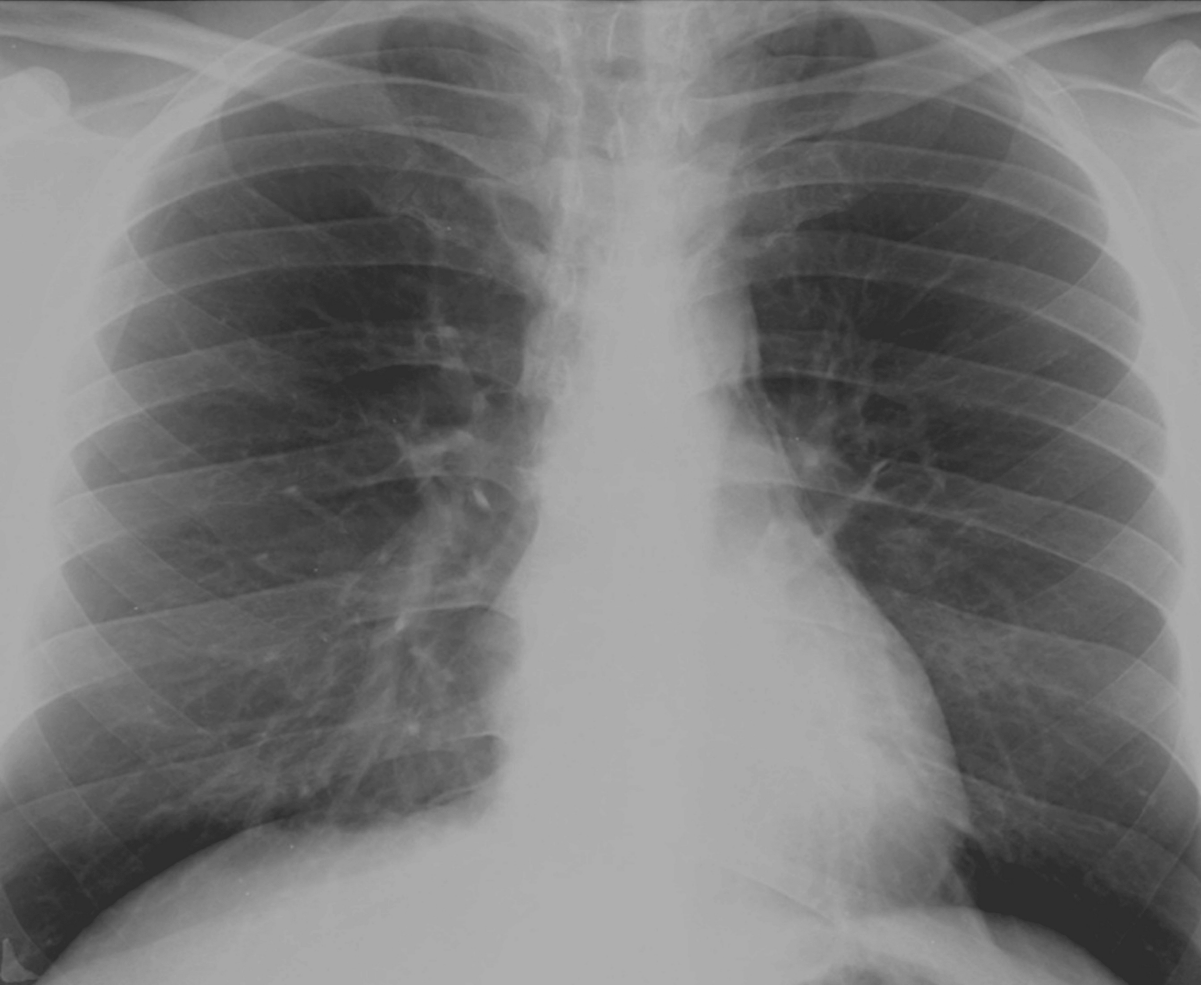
Postoperative fifth day complete regression of pneumomediastinum seen on chest X-ray
